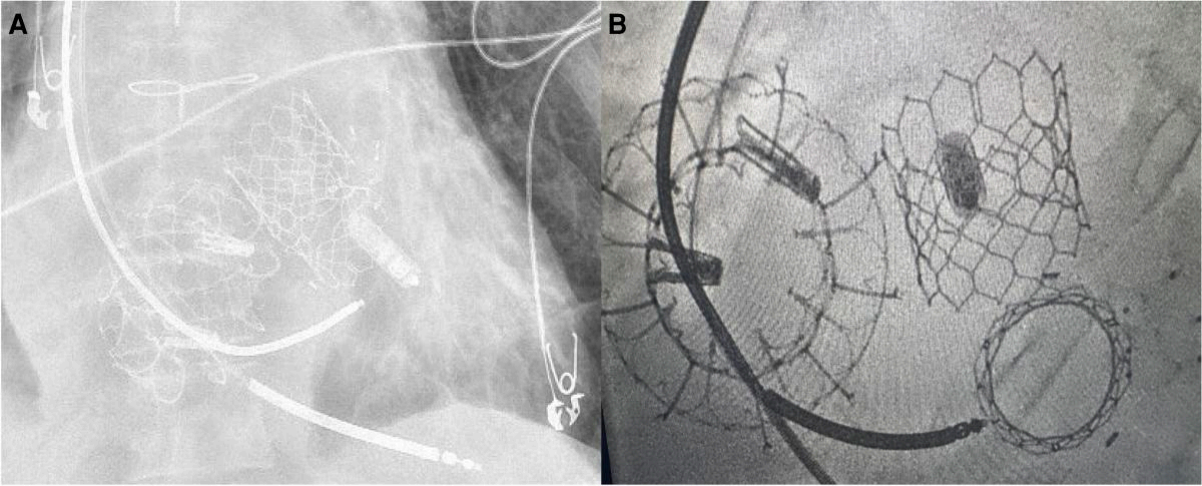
(*A*) Chest X-ray image of Sapien TAVR, EVOQUE TTVR-in-Triclip, and Sapien TMVR-in-valve; (*B*) fluoroscopic image of Sapien TAVR, EVOQUE TTVR-in-Triclip, and Sapien TMVR-in-valve with prior paravalvular leak occluders for paravalvular surgical valve leak.
